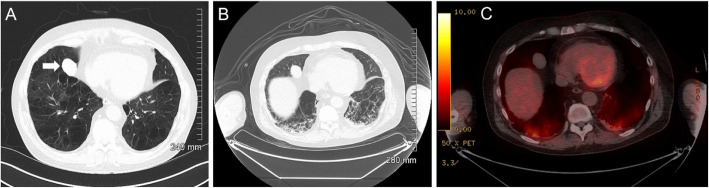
Initial CT thorax detected a right middle lobe mass (a). Subsequent PET-CT demonstrated no FDG uptake b (CT) and c (fused PET-CT)
